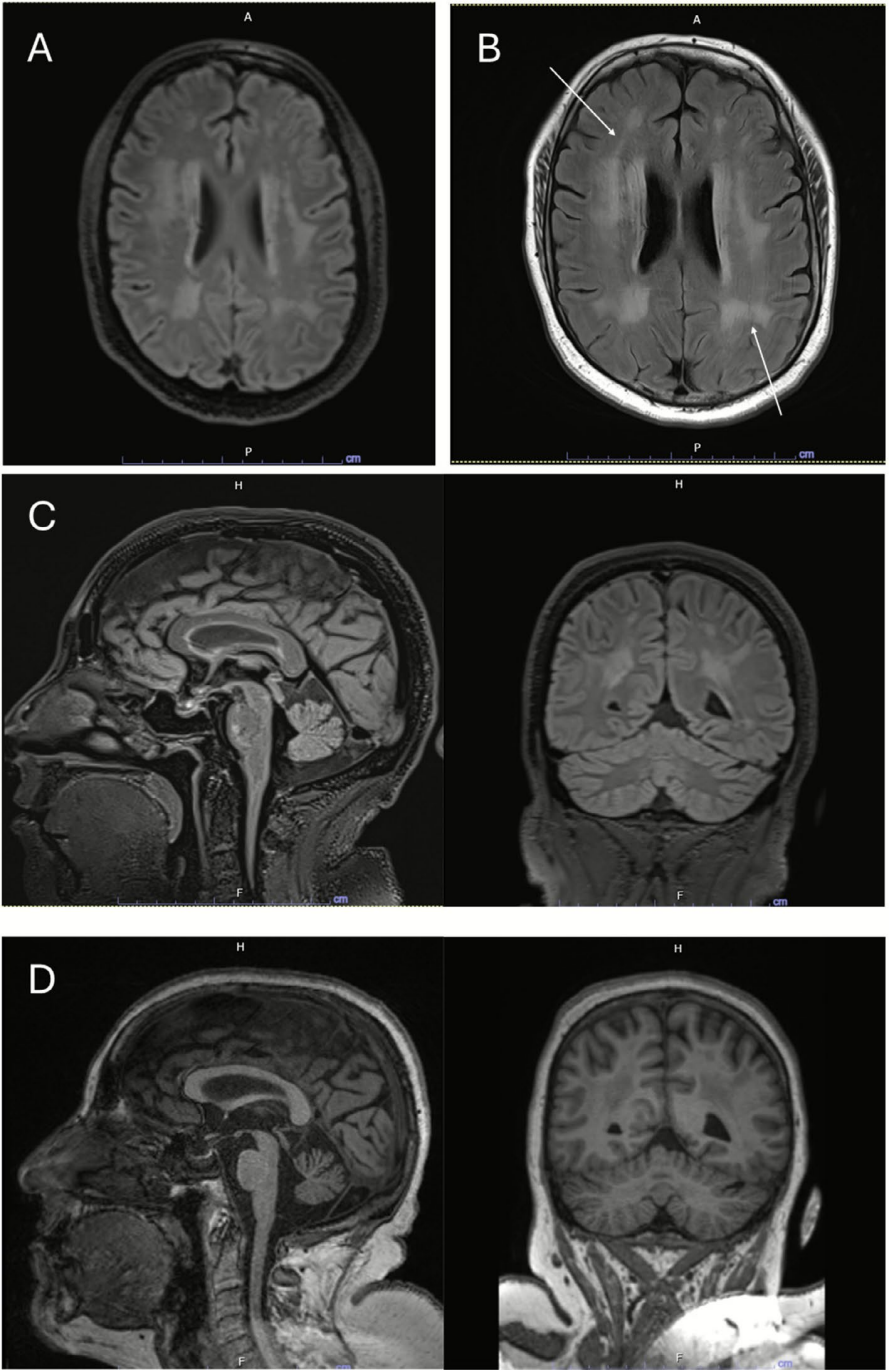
MRI demonstrating subtle progressive leukodystrophy and cerebellar atrophy. (A) and (B) are single axial slices of fluid attenuated inversion recovery (FLAIR) sequences from the initial MRI following liver transplantation (A) and the most recent follow‐up imaging in 2024 (B). Arrows demonstrate progressive confluence of periventricular T2 hyperintensities. (C) and (D) are sagittal (left) and coronal (right) slices from FLAIR sequence for the initial MRI following liver transplantation (C) and T1 weighted images from the most recent follow‐up imaging in 2024 (D). There is possible increased prominence of cisternal and subarachnoid spaces in the most recent images. Due to the transition of care there were no comparable imaging sequences to compare cerebellar size.
